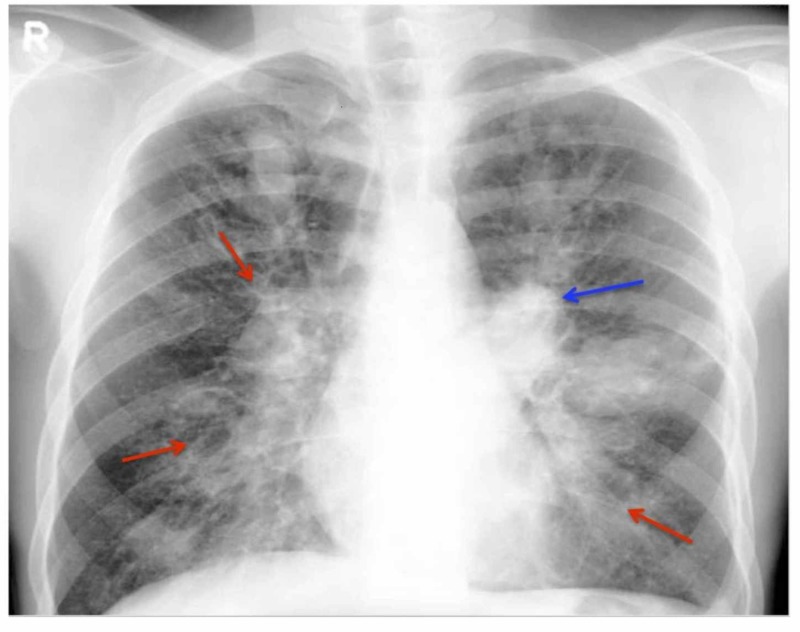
Plain Chest Radiograph The chest radiograph of an allergic bronchopulmonary aspergillosis (ABPA) patient shown with left-sided perihilar opacity (blue arrow) along with non-homogeneous infiltrates (transient pulmonary infiltrates indicated by red arrows) in all zones of both lung fields, seen in acute and remission stage of ABPA [3].
